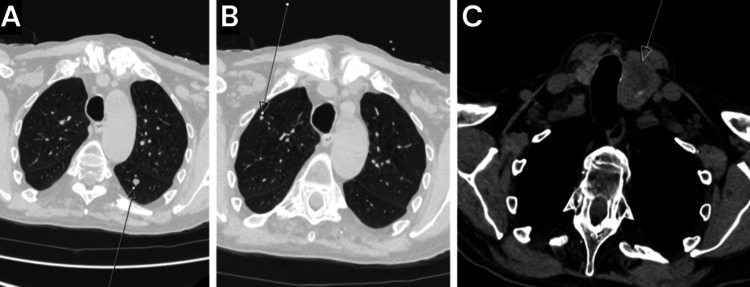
Contrast-enhanced CT of the aorta showing pulmonary and thyroid lesions consistent with metastatic disease (A) Axial CT view demonstrating a 6.1 mm pulmonary nodule in the left lower lobe, along with multiple other bilateral pulmonary nodules likely representing metastases (arrow). (B) Axial CT view showing a 5 mm pulmonary nodule in the right lung apex (arrow). (C) Axial CT view showing an enlarged left thyroid lobe with multiple nodules (arrow).
